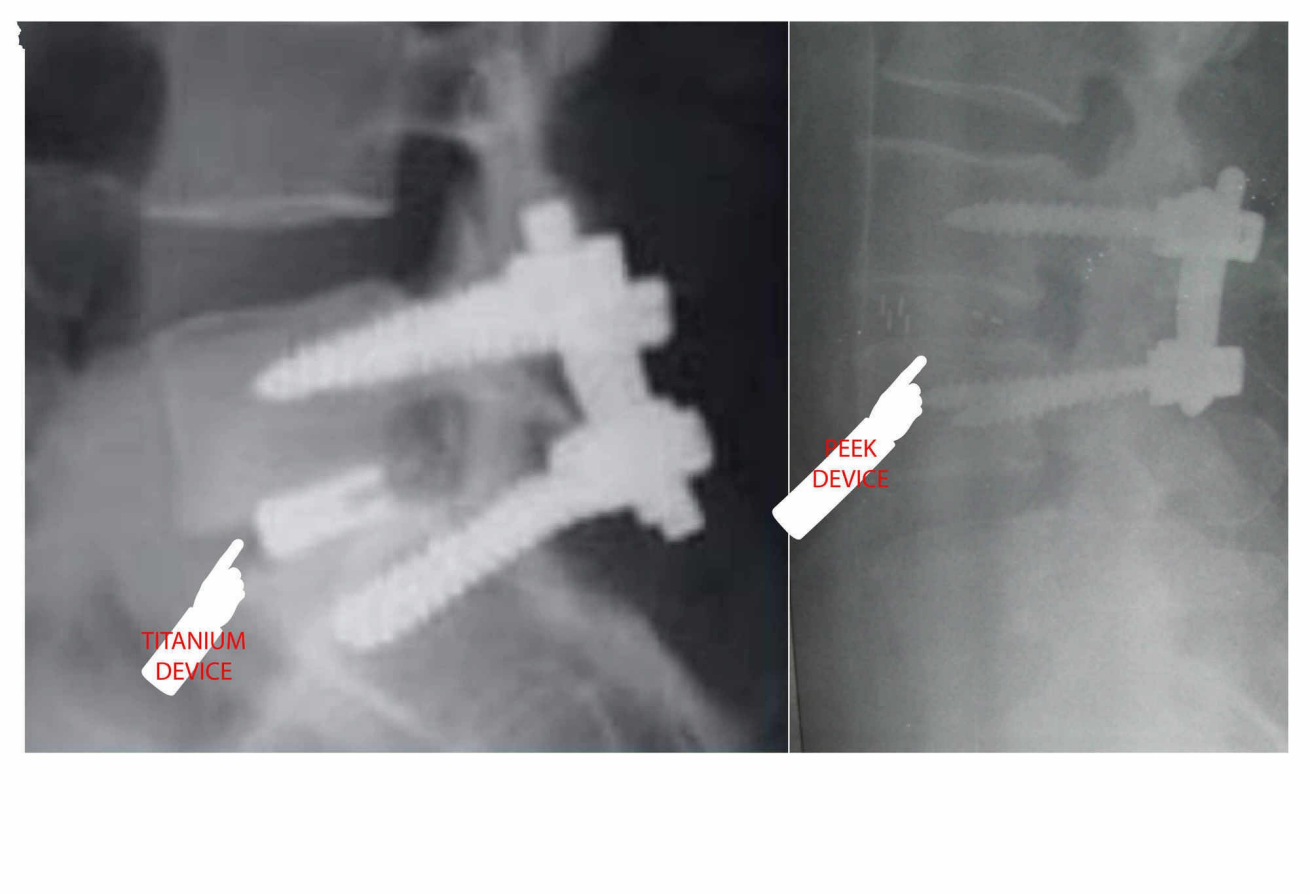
Radiography picture from a circumferential spine fusion with titanium (picture on the left) and PEEK (picture on the right) PEEK: polyester ether ketone
